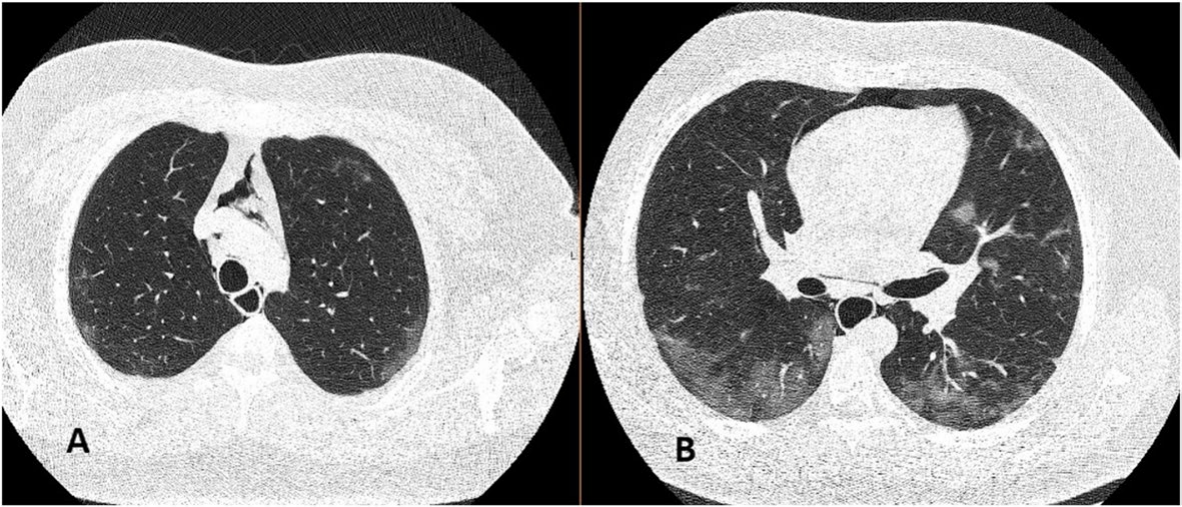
Chest CT obtained at presentation to the ED in case 2, demonstrating mediastinal air (A). It shows bilateral ground-glass opacities and parenchymal consolidation (A–B)
